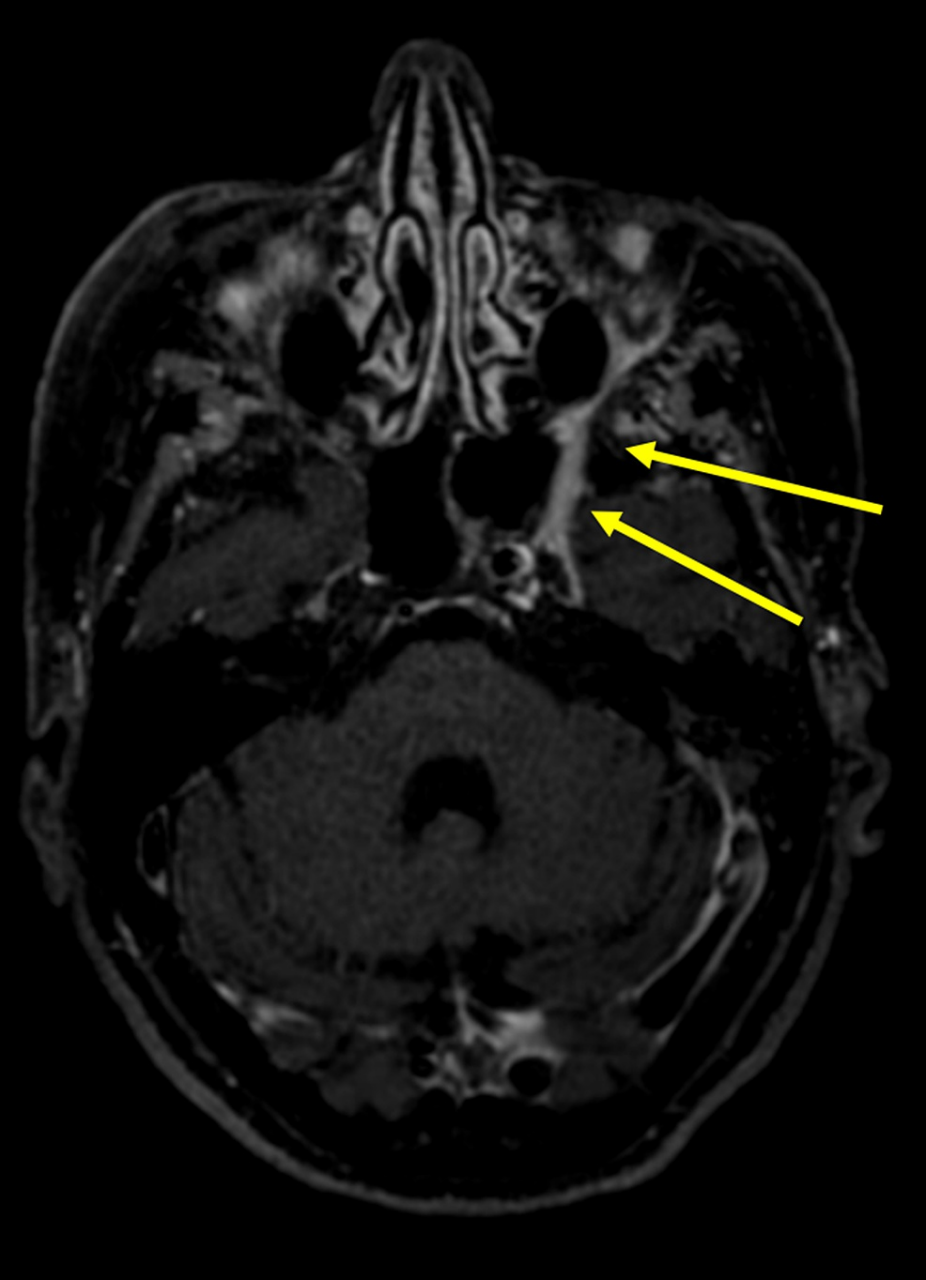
Gadolinium-enhanced axial post-contrast T1-weighted 3-Tesla MRI images of the brain showing perineural tumor spread (yellow arrows).
